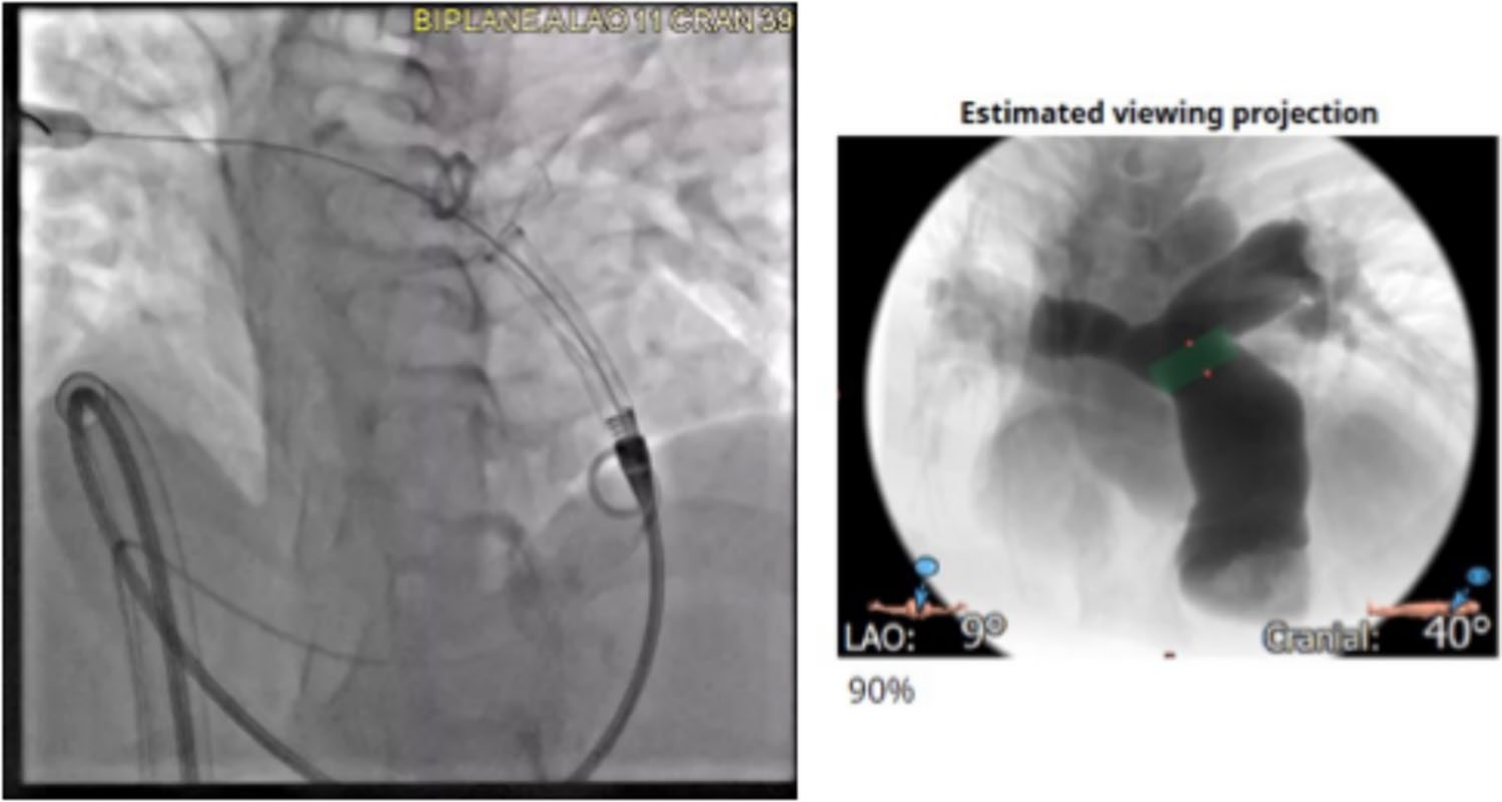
Shows the estimated optimal angiographic projection using the CT images on the right. The estimated distal landing zone shown in green. On the left panel, the actual angiographic deployment is shown taking benefit from CT-based predictions
